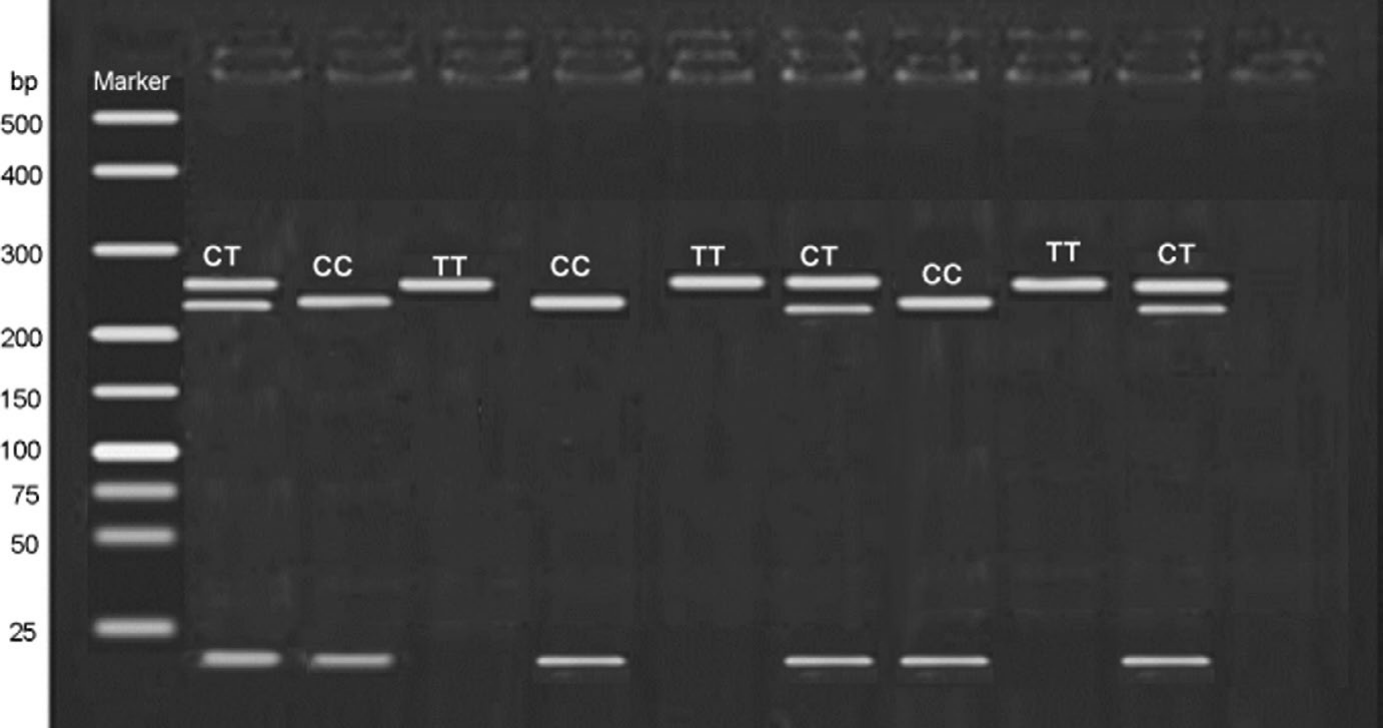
The digestion results for rs1861494 polymorphism. The amplified production of rs1861494 polymorphism was digested by *Msp*I, and the results were as follows: TT (267bp), CT (267bp, 245bp and 22bp) and CC (245bp and 22bp)
